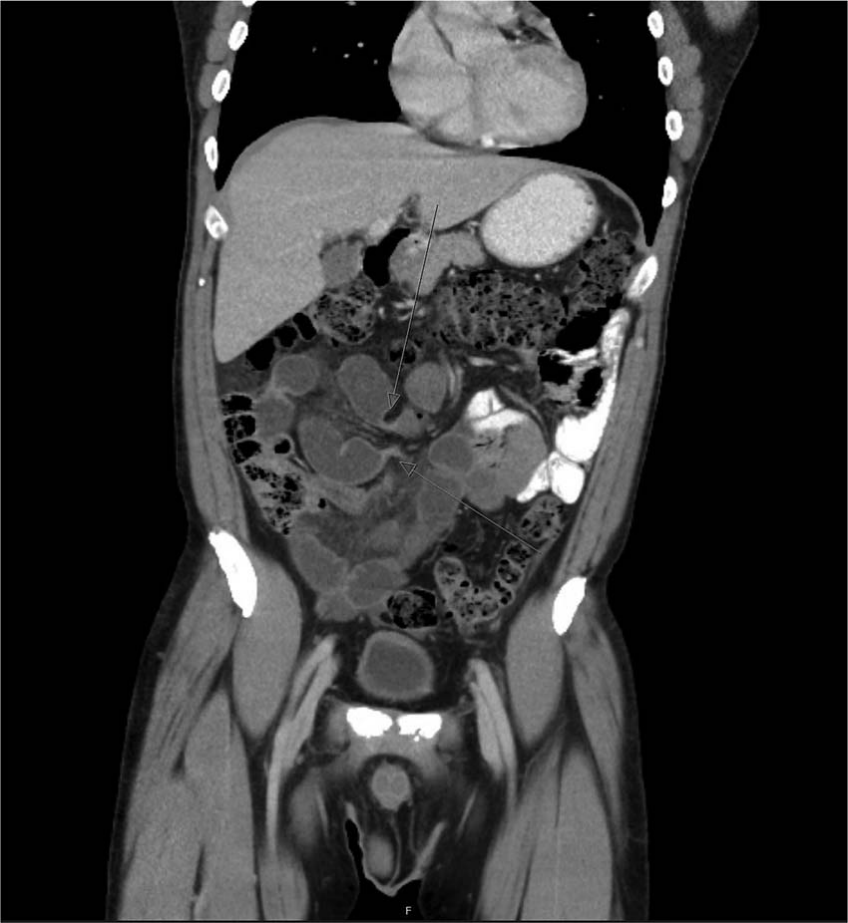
Abdominal CT scan demonstrating closed-loop small bowel obstruction involving jejunal loops, with two distinct transition points indicated by arrows.
